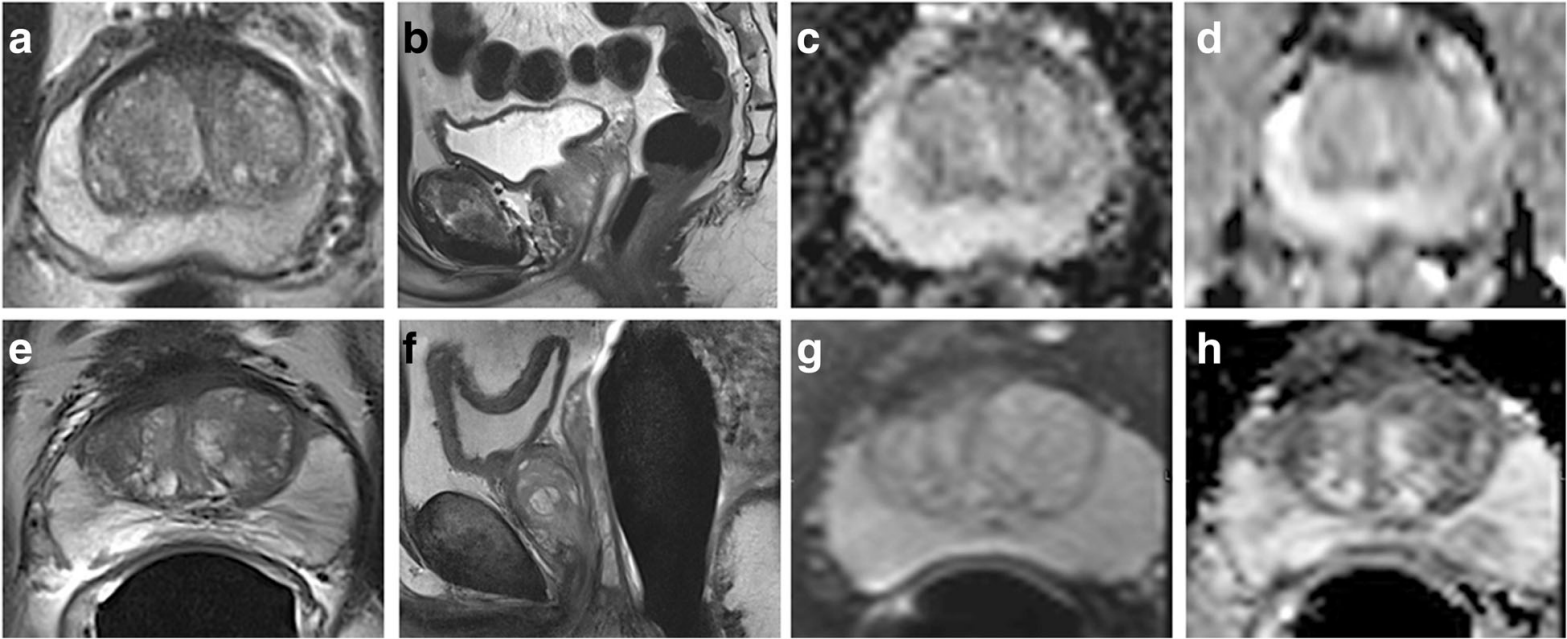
Biparametric magnetic resonance imaging at 3.0 T in patients with benign prostate hyperplasia was performed without endorectal coil at site 1 (a–d) and with endorectal coil at site 2 (e–h) included in a multichannel phased-array coil system: axial (a, e) and sagittal (b, f) T2-weighted imaging, axial diffusion-weighted imaging with a *b* value of 800 s/mm^2^ (c, g), and corresponding apparent diffusion coefficient map (d, h) obtained with a reduced field of view
